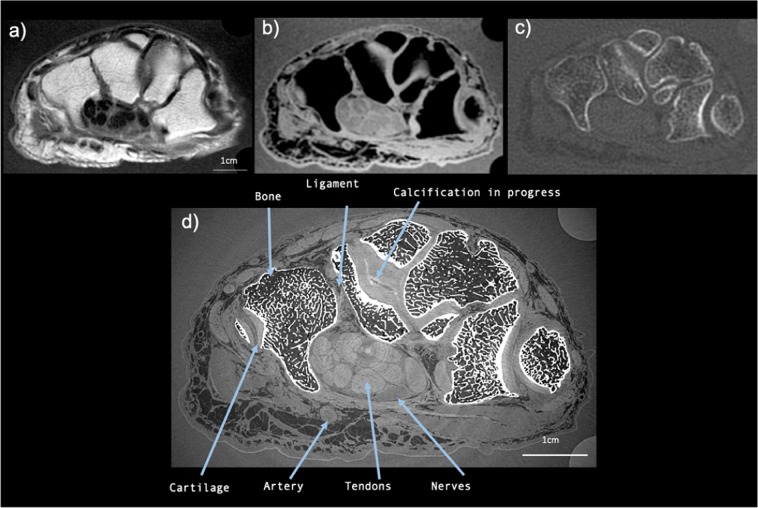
PCI-CT and conventional imaging modalities applied to the same hand area. Images obtained with MRI (a) T1W SE, (d) (b) 3D WATSC, (c) CT with bone windowing and (d) PCI-CT.
